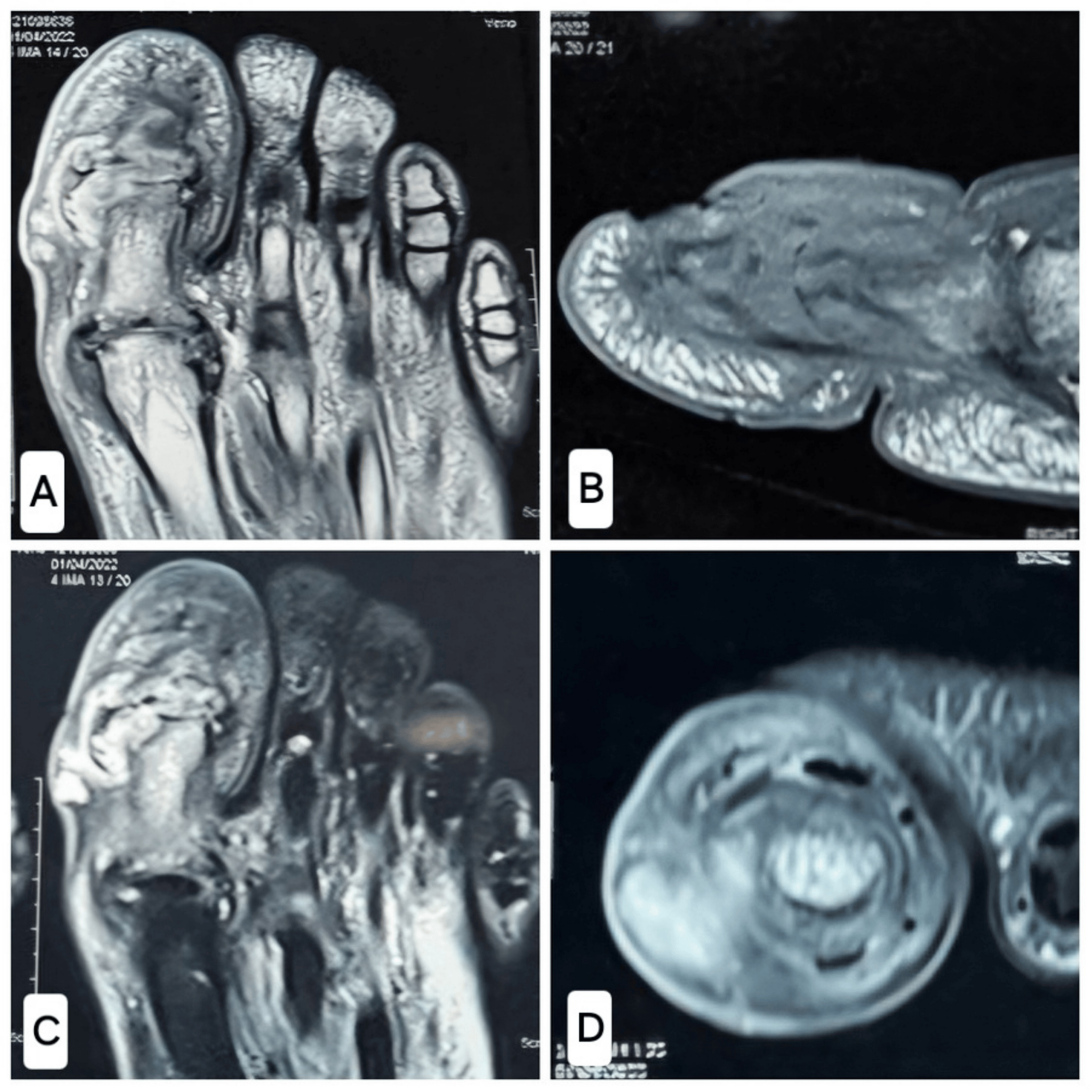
MRI of the affected great toe. A, B: T1 images of the affected great toe. C, D: T2 images of the affected great toe.
